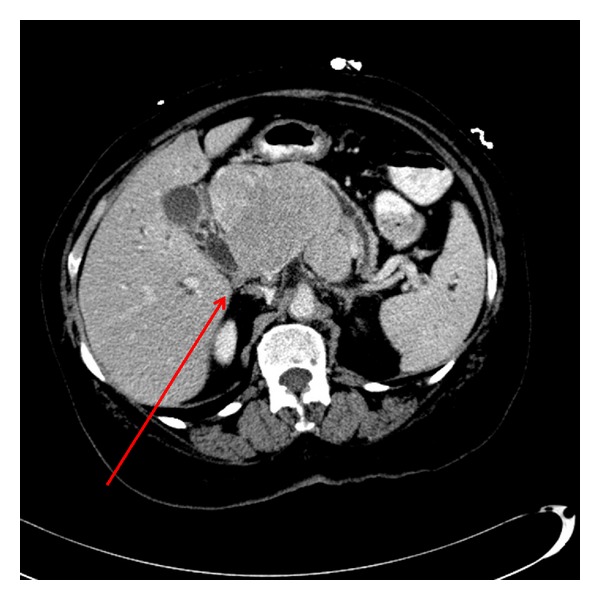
Contrast-enhanced axial CT image demonstrates a large homogeneously enhancing mass in the region of the pancreatic head which is causing compression of the distal common bile duct (red arrow).
